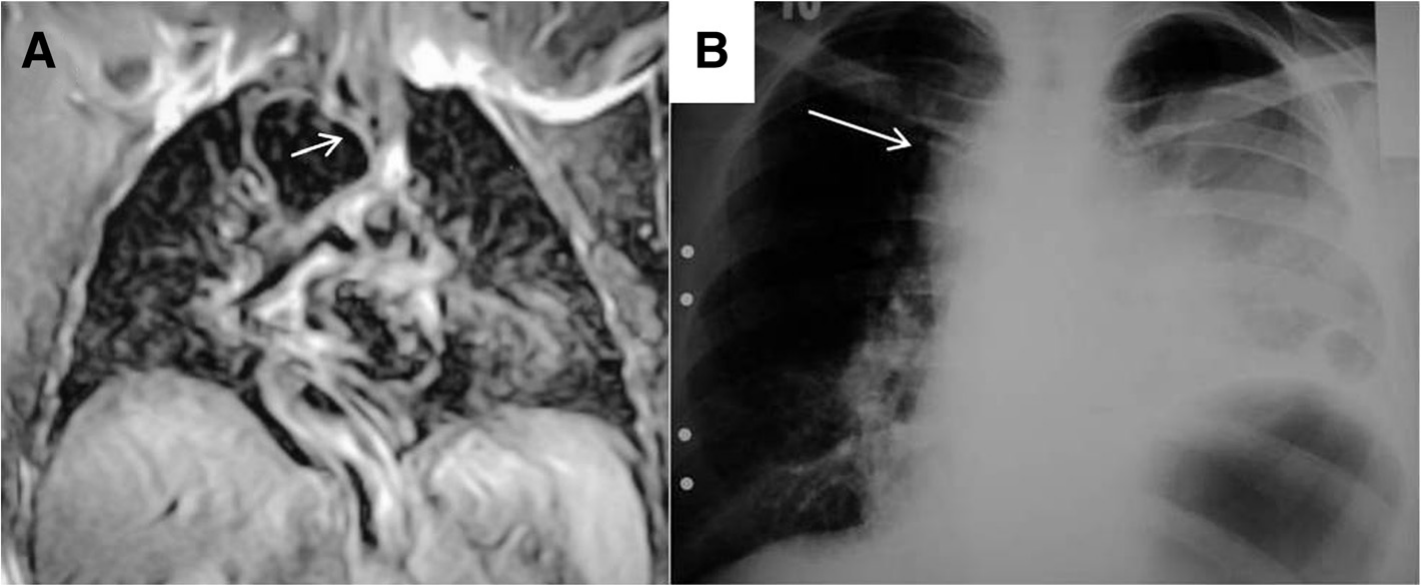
Thoracic MRI and Chest radiograph images; marked compression of the trachea is seen on thoracic MRI (5A). A chest radiograph showed a lung cancer in the left hemithorax and right sided arcus aorta (5B, white arrow).
